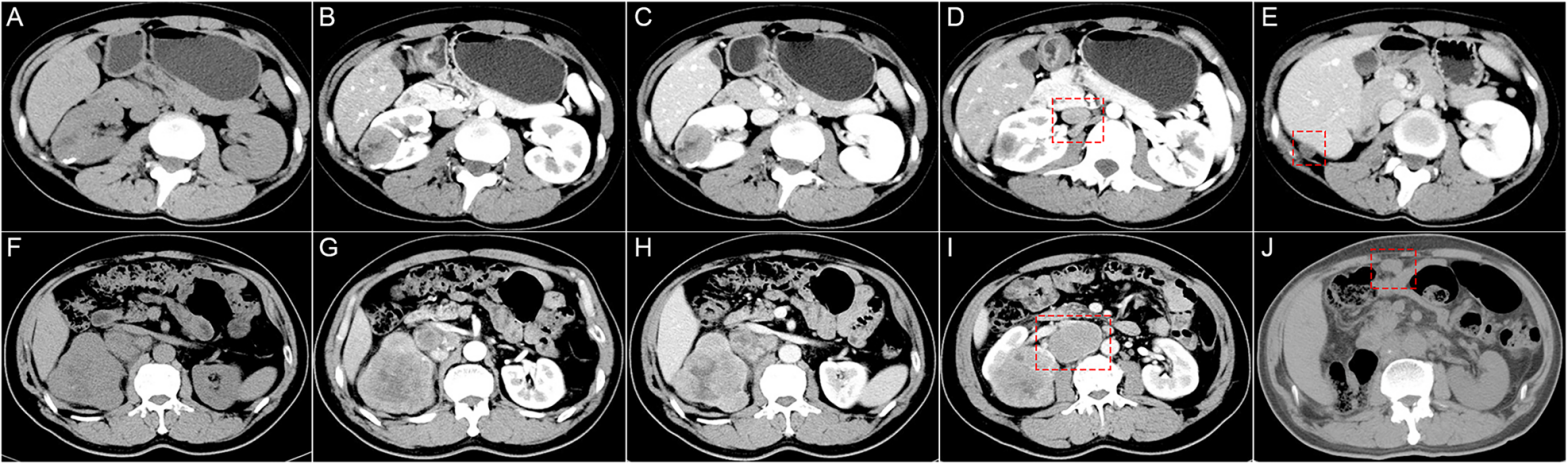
Preoperative axial contrast-enhanced CT images, from left to right: pre-contrast phase, corticomedullary phase, nephrographic phase, regional lymph node display, and post-surgery CT follow-up. A–E A 23-year-old female patient diagnosed with papillary renal cell carcinoma (PRCC) underwent radical nephrectomy. The tumor measured approximately 3.4 cm, with a tumor margin regularity score of 1 point. The short diameter of the right renal hilar lymph node was 8 mm, corresponding to a regional lymph node score of 1 point. The patient was classified as high-risk (Radiological score = 2). Subcapsular liver nodules were identified on follow-up CT 17.5 months post-surgery, which were subsequently confirmed as a recurrence of PRCC through surgical pathology. F–J A 58-year-old male patient diagnosed with PRCC underwent radical nephrectomy and lymph node dissection. The tumor measured approximately 9 cm, with a tumor margin regularity score of 1 point. The short diameter of the right renal hilar lymph node was 38 mm, corresponding to a regional lymph node score of 2 points. The patient was classified as high-risk (Radiological score = 3). Intra-abdominal nodule was identified on follow-up CT 3 months post-surgery, which was subsequently confirmed as a recurrence of PRCC through surgical pathology
